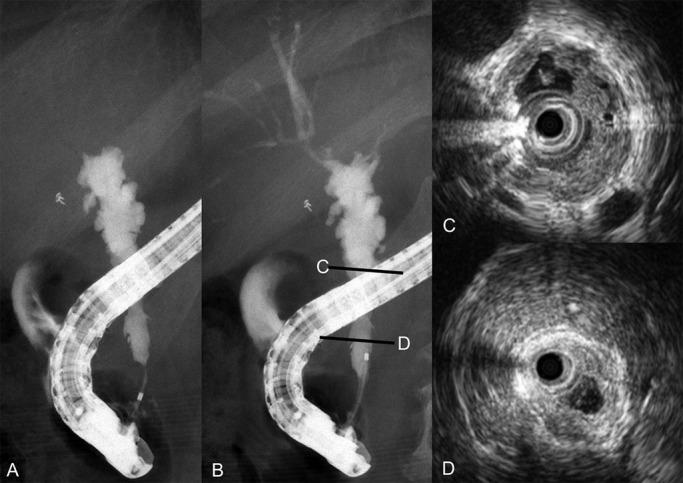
Endoscopic retrograde cholangiography and intraductal ultrasonography findings after clinical improvement. A–B) Endoscopic retrograde cholangiography revealed stenosis and outpouching. C–D) Intraductal ultrasonography depicted inhomogeneous wall thickness in the mid-portion of the common bile duct.
